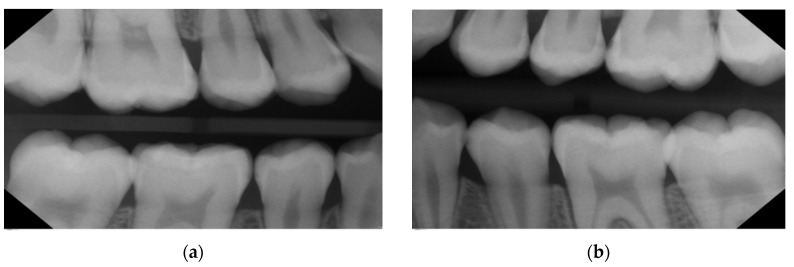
Case 1—Baseline in 11/2019: Bitewings show multiple proximal lesions but without clear cavitation on the right (a) and left side (b). The patient was 13 years old and had a history of fixed orthodontic appliances. Only non-invasive caries management options were undertaken, as due to COVID-19 pandemic, the patient did not show up for micro-invasive or restorative treatment until 2022—see Figure 2. For a Lesion assessment, see Table 1.
